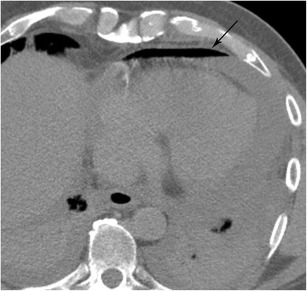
Pericardioesophageal Fistula. CT of the chest in soft tissue window demonstrates fluid and gas (arrow) within the pericardium in a patient with a confirmed pericardioesophageal fistula
